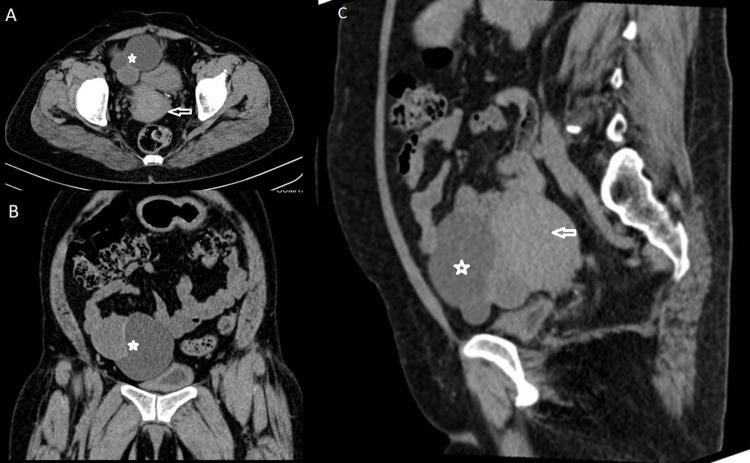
Case 1 CT transverse section (A), coronal section (B), and sagittal section (C) reformations showing multiloculated cystic lesion (marked with asterisks) anterolateral to the uterus (arrows in A & C) on right side
